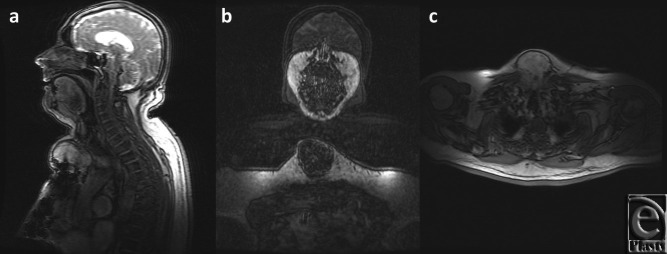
Magnetic resonance images in sagittal (*a*), frontal (*b*), and transverse (*c*) planes of the granulation arising from the upper sternum. The granulation tissue is seen extending anteriorly and posteriorly on the sagittal and transverse planes.
